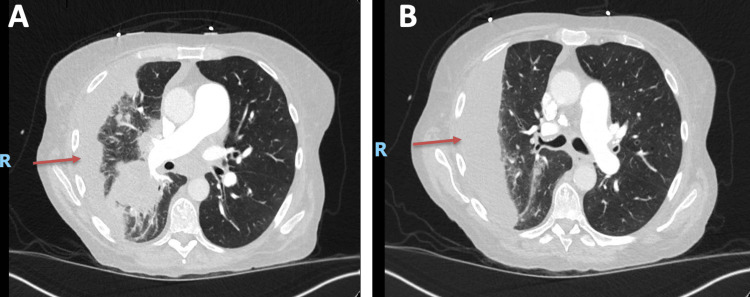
(A, B) Computed tomography of the chest with contrast on admission: consolidation involving the right middle and lower lobes and moderate-sized right partially loculated pleural effusion
